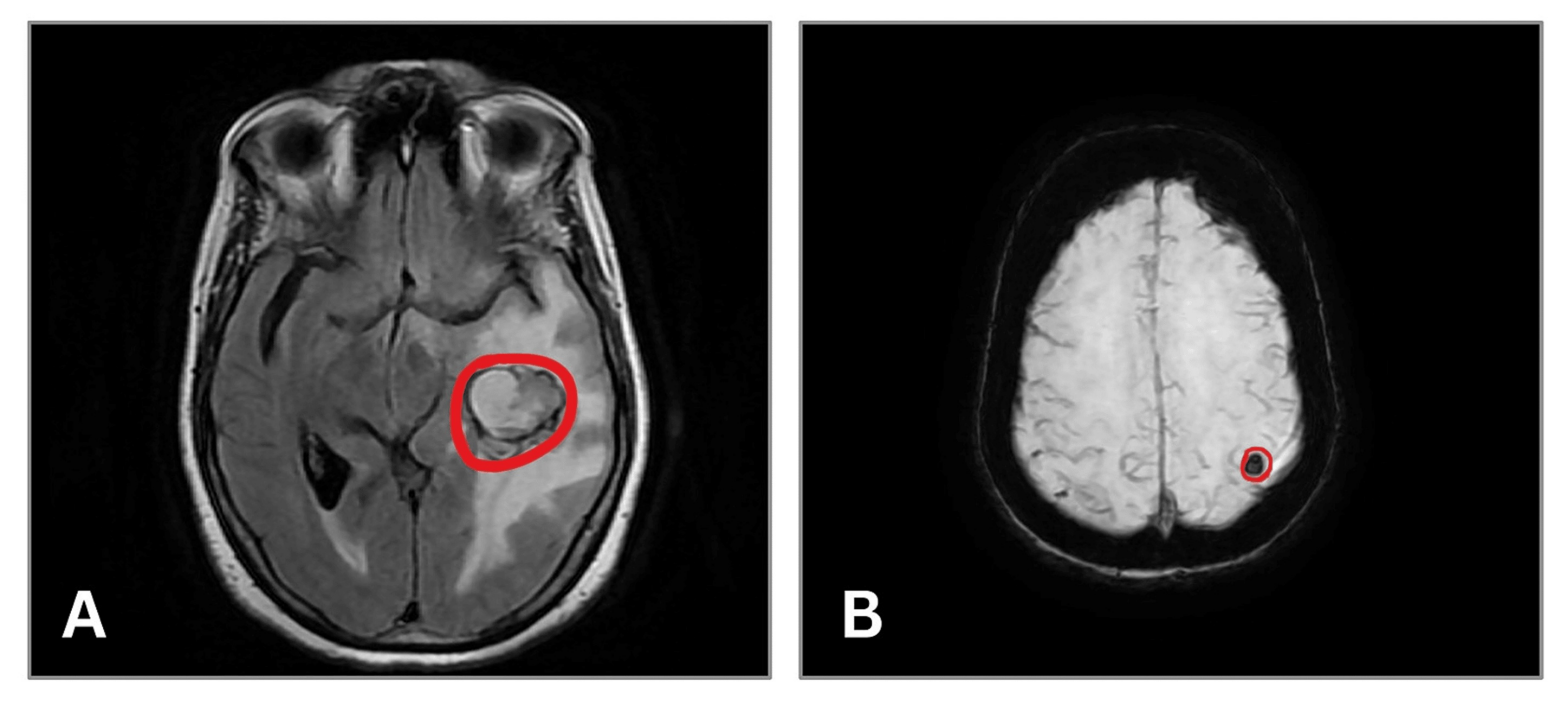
Magnetic resonance imaging (MRI) of the brain demonstrating intracranial lesions (A)  Axial diffusion-weighted image demonstrating a well-defined lobulated solid-cystic lesion in the left temporal lobe with diffusion restriction and surrounding perilesional edema (circled). (B) Axial image demonstrating nodular lesion in the left parietal lobe showing blooming on susceptibility-weighted imaging (SWI) with disproportionate perilesional edema (circled).
